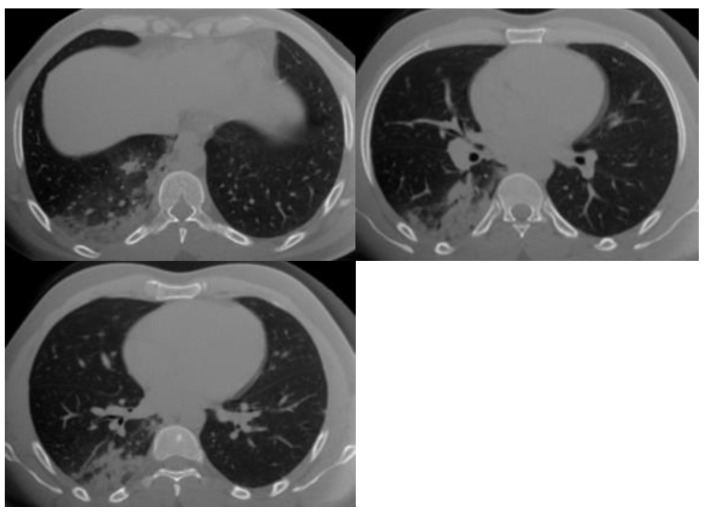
Multiple chest CT images of COVID-19 patients. Two senior radiologists, a pulmonologist, and a house officer agreed to calculate the CO-RADS for this case as CO-RADS 4. Agreement was based on the following features: multifocal unilateral GGO and consolidation close to the pleura. One junior radiologist with another house officer agreed to calculate the CO-RADS for this case as CO-RADS 5. The agreement was based on the following features: multifocal unilateral GGO, consolidation close to the pleura, and other side unifocal GGO.
